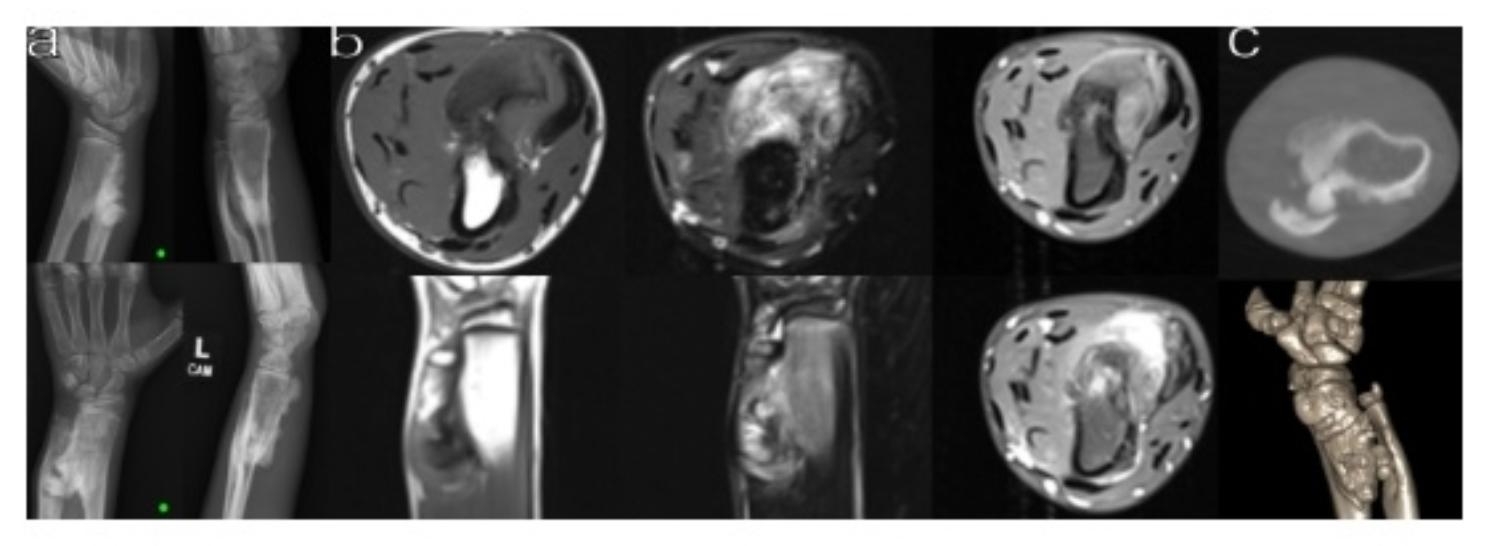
a-c: Preoperative imaging. a Radiographs of the left wrist demonstrating the parosteal osteosarcoma. The two top radiographs are the original images taken before the patient was lost to follow-up. The bottom set are one year later. b MR imaging left to right show the heterogeneous T2 hyperintense and T1 isointense to hypointense, avidly enhancing exophytic cortical medullary mass emanating from the ulnar aspect of the radial metadiaphysis extending locally into the adjacent ulna. c CT three dimensional reconstruction used for operative planning
